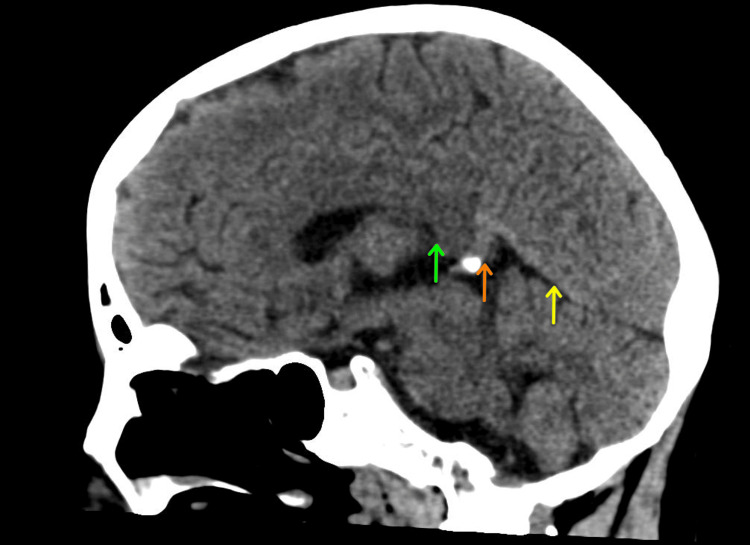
Sagittal non-contrast CT of the brain post-treatment showing complete resolution of the previously observed thrombosis in the internal cerebral veins (green arrow), the vein of Galen (orange arrow), and straight sinus (yellow arrow).
